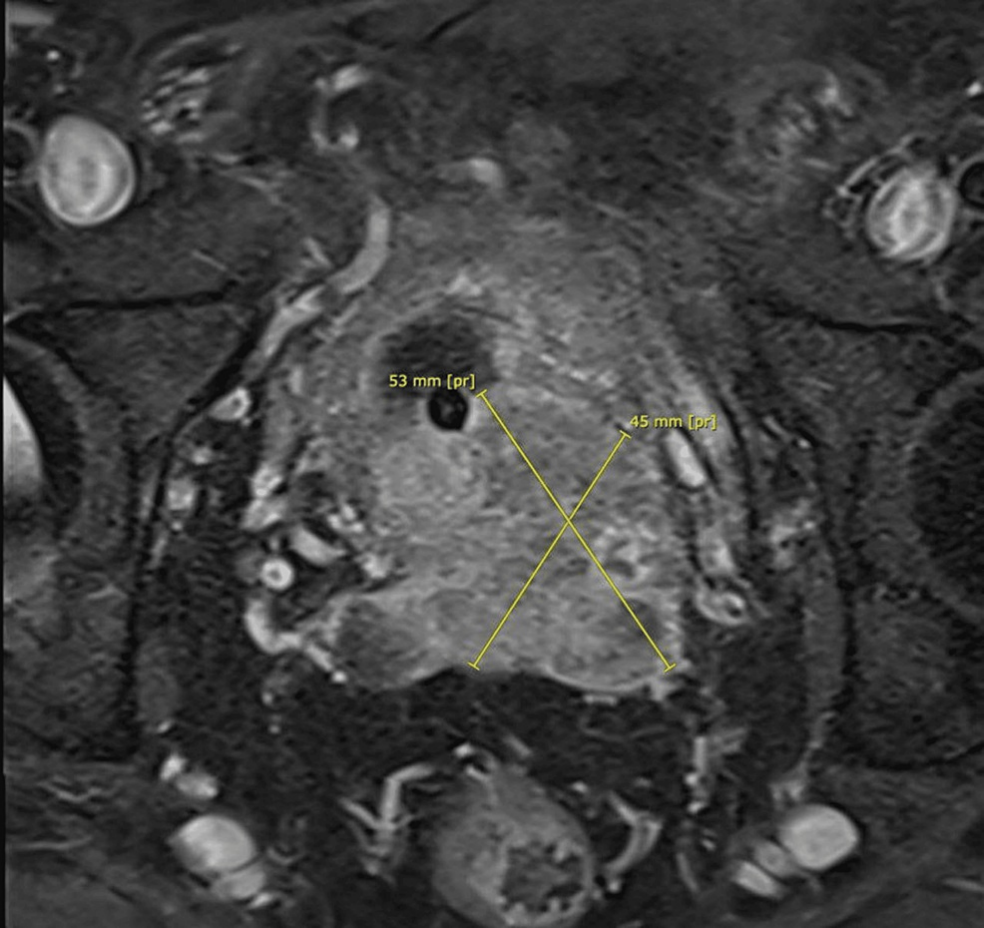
MRI of the prostate with contrast showing PI-RADS5 prostate lesion Diffusely enlarged prostate measuring 5.5 × 4.5 × 6.5 cm, with a volume of 80 g, demonstrates heterogeneous ill-defined lobulated abnormal signal intensity lesion about 5 cm in diameter of moderate T2 homogeneous hypointensity involving mainly the transition zone, extending to the bladder base, seminal vesicles, extra-prostatic left posterolateral neurovascular bundle, and abutting left levator ani, mainly involving the left side of the prostatic base MRI, magnetic resonance imaging; PI-RADS5, Prostate Imaging-Reporting and Data System 5
